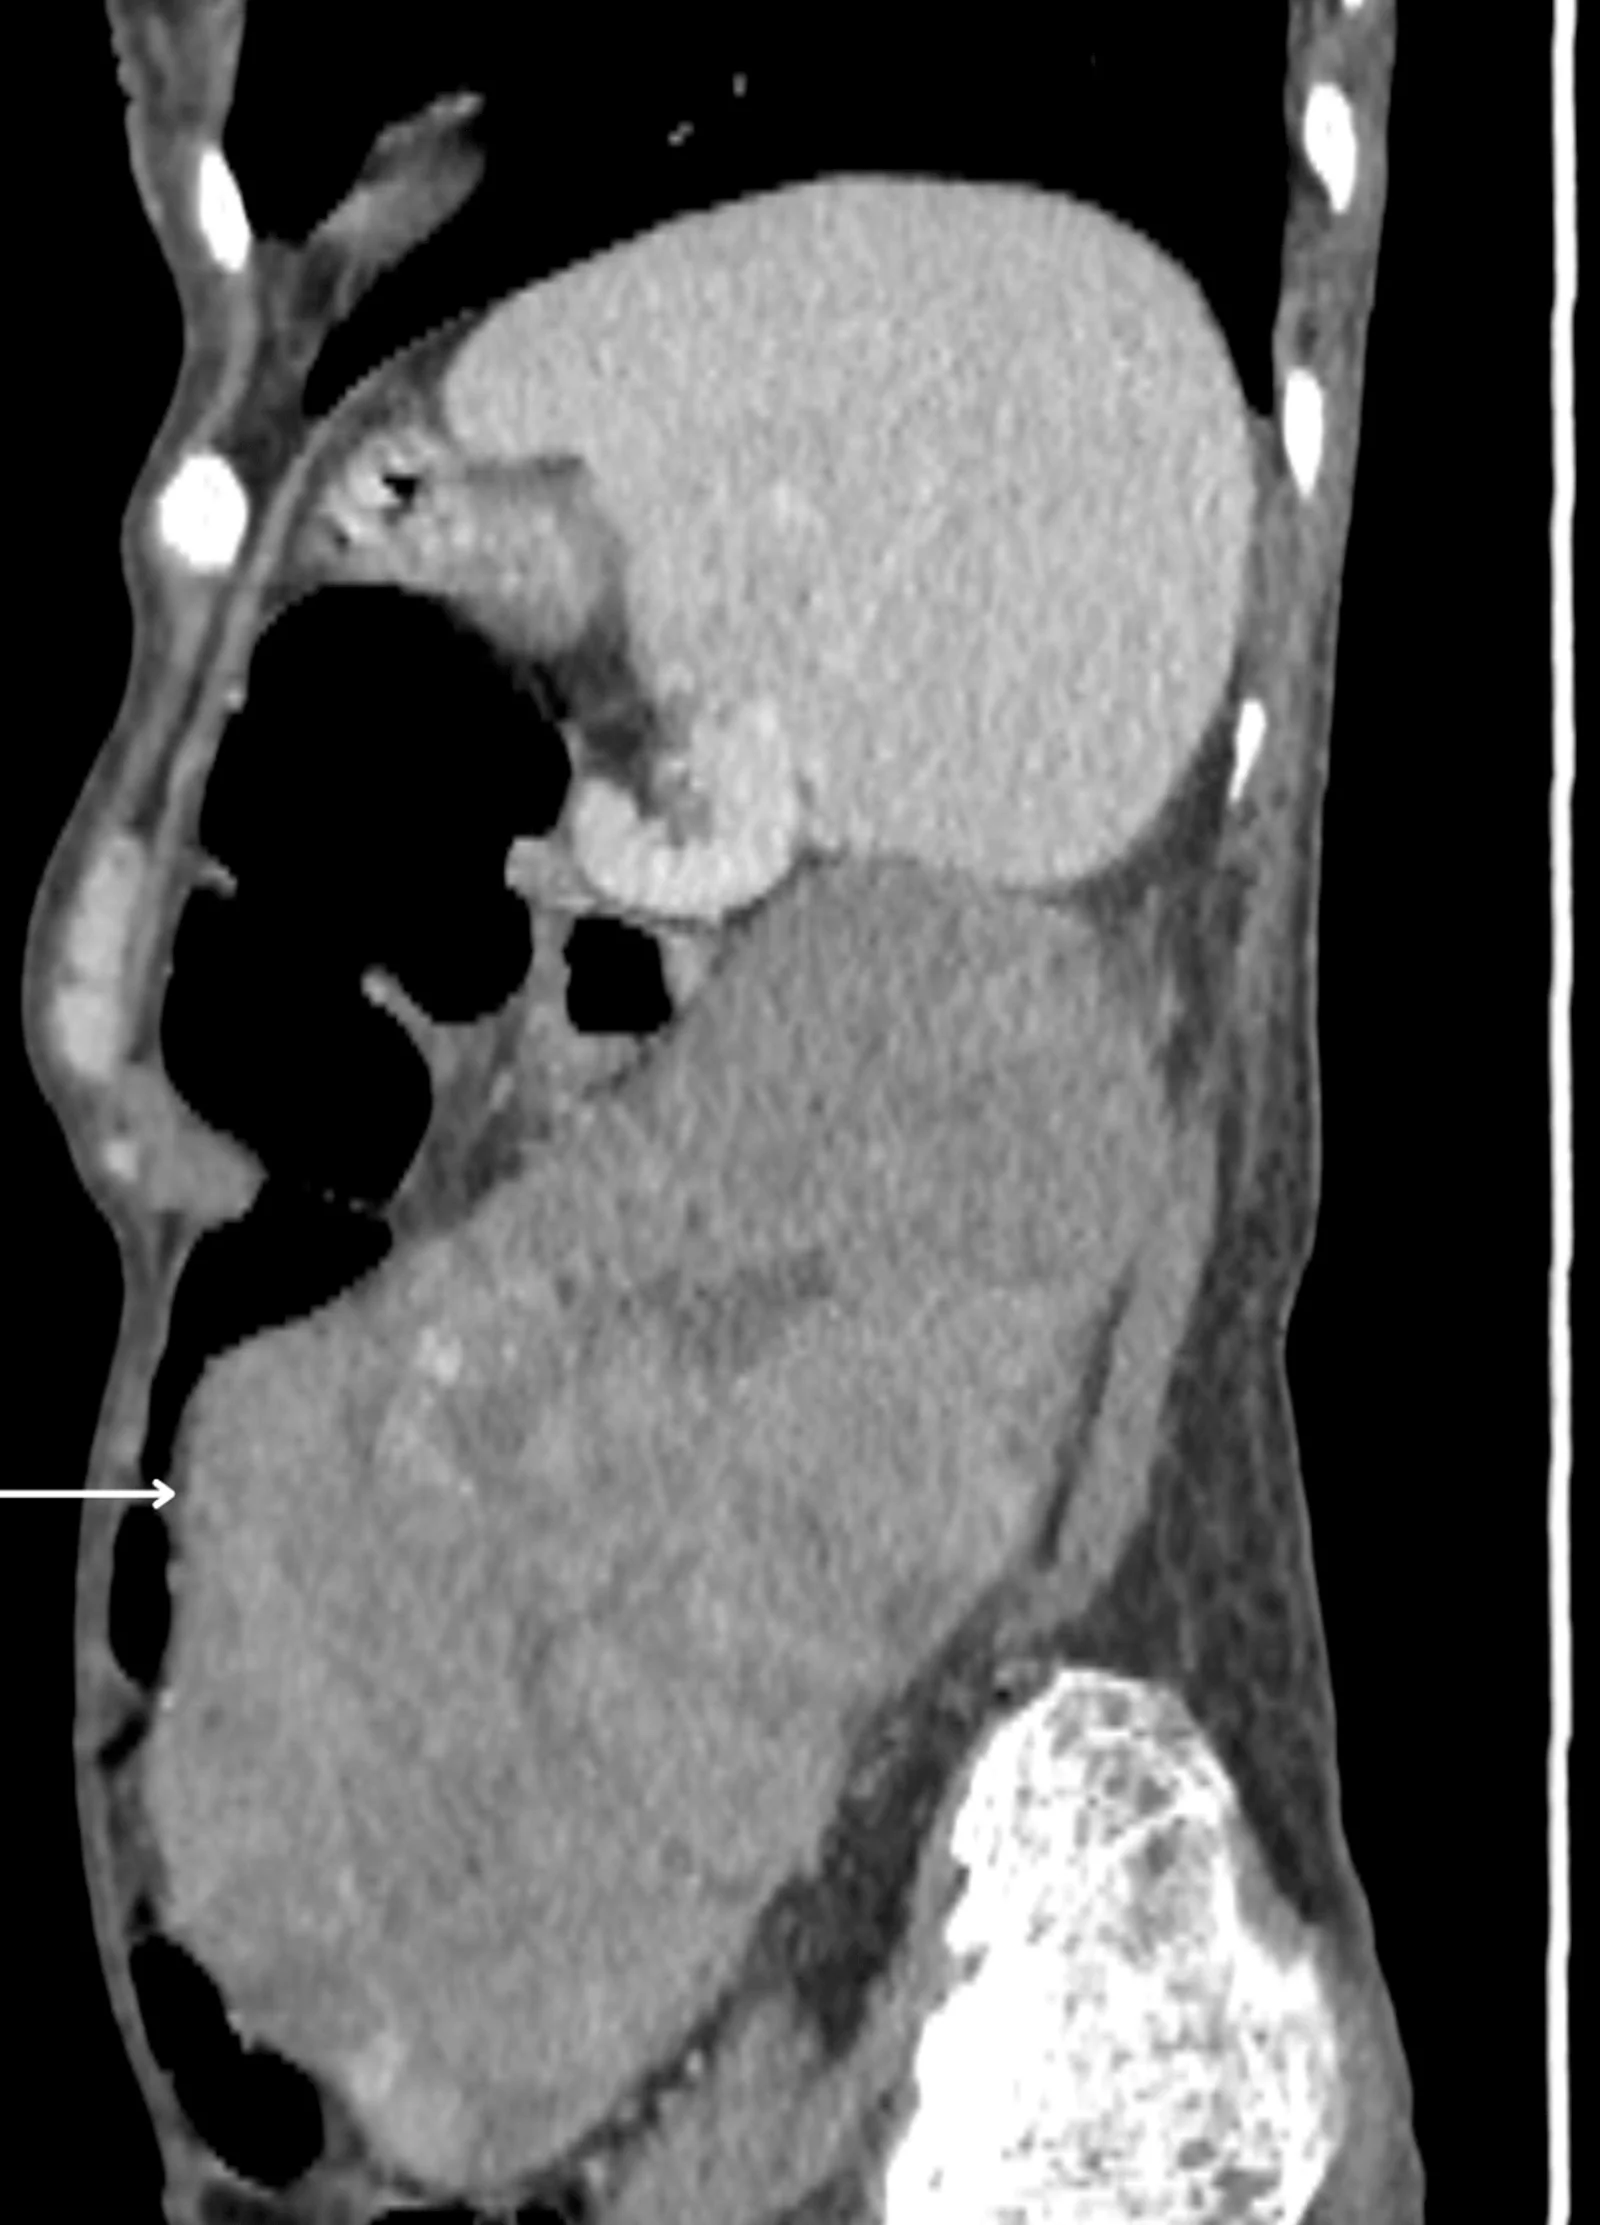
Sagittal contrast-enhanced CT image demonstrating a giant heterogeneous left renal mass with extensive central necrosis and marked mass effect on adjacent abdominal structures (arrow).
